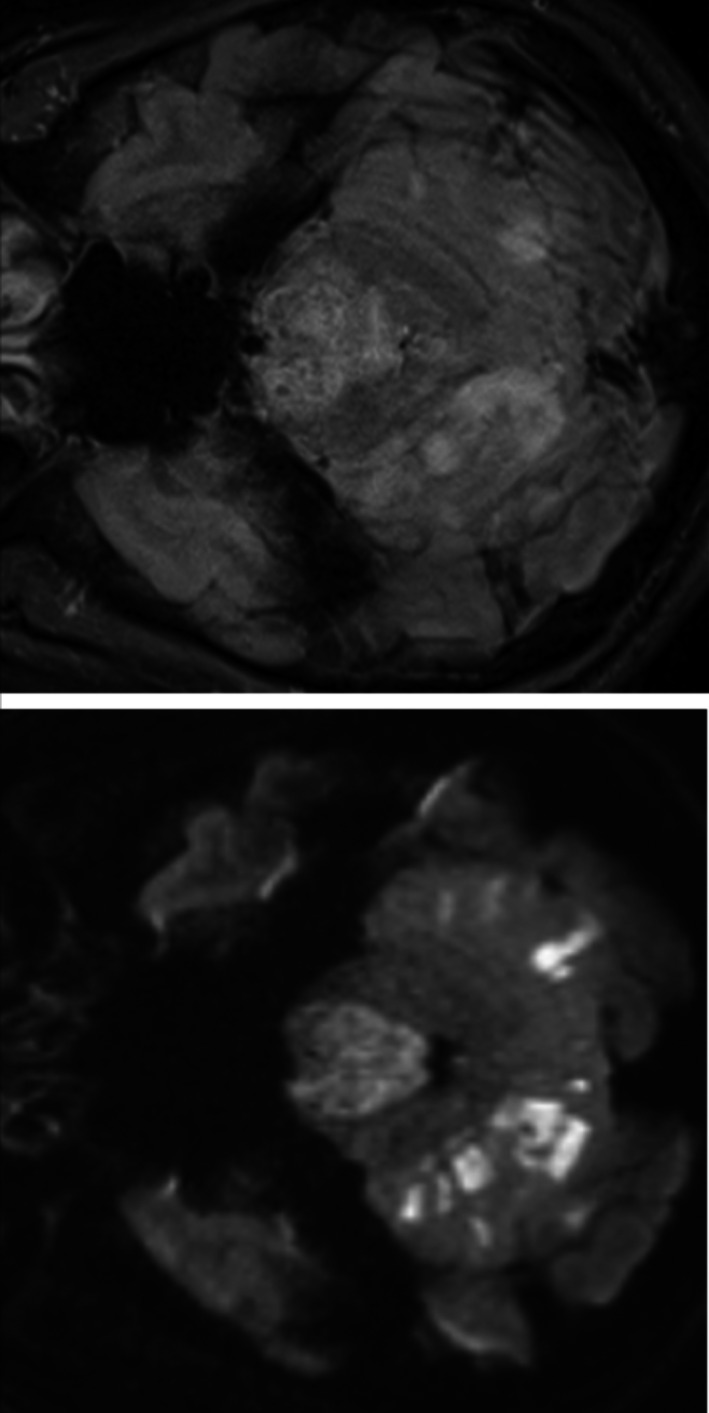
Twenty‐four hours post‐thrombectomy MRI: DWI (left) and fluid‐attenuated inversion recovery (FLAIR) (right) showing no increased area of pontine and cerebellar infarction, but more intense restricted diffusion with better defined lesion
